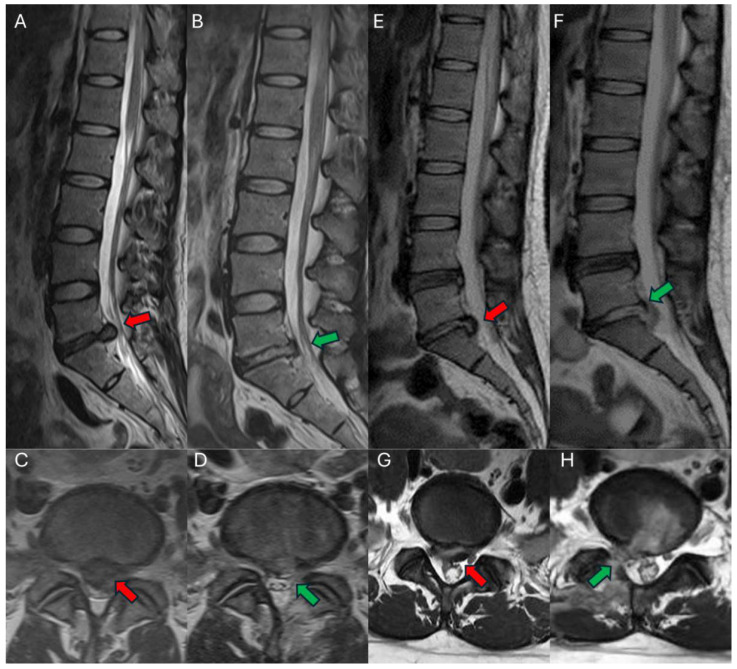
(A–D) Case 1: 35-year-old male. (E–H) Case 2: 39-year-old female. Red arrows indicate preoperative MR images; green arrows indicate postoperative MR images following PEID.
